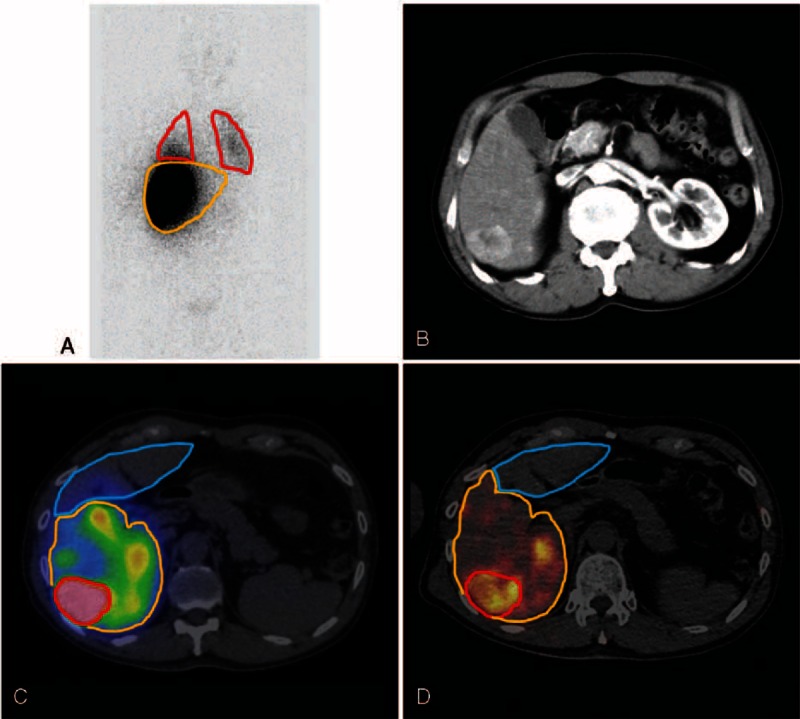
ROIs and VOIs for image analysis. LSF was measured on anterior and posterior ^99m^Tc-MAA planar scans. ROIs are drawn on the lungs (red) and liver (yellow) (A). With reference to contrast-enhanced CT images (B), VOIs for 3 partitions of tumor (red), in-target normal liver (yellow), and out-target normal liver (blue) were drawn on ^99m^Tc-MAA SPECT/CT (C) and ^90^Y-microsphere PET/CT (D).
